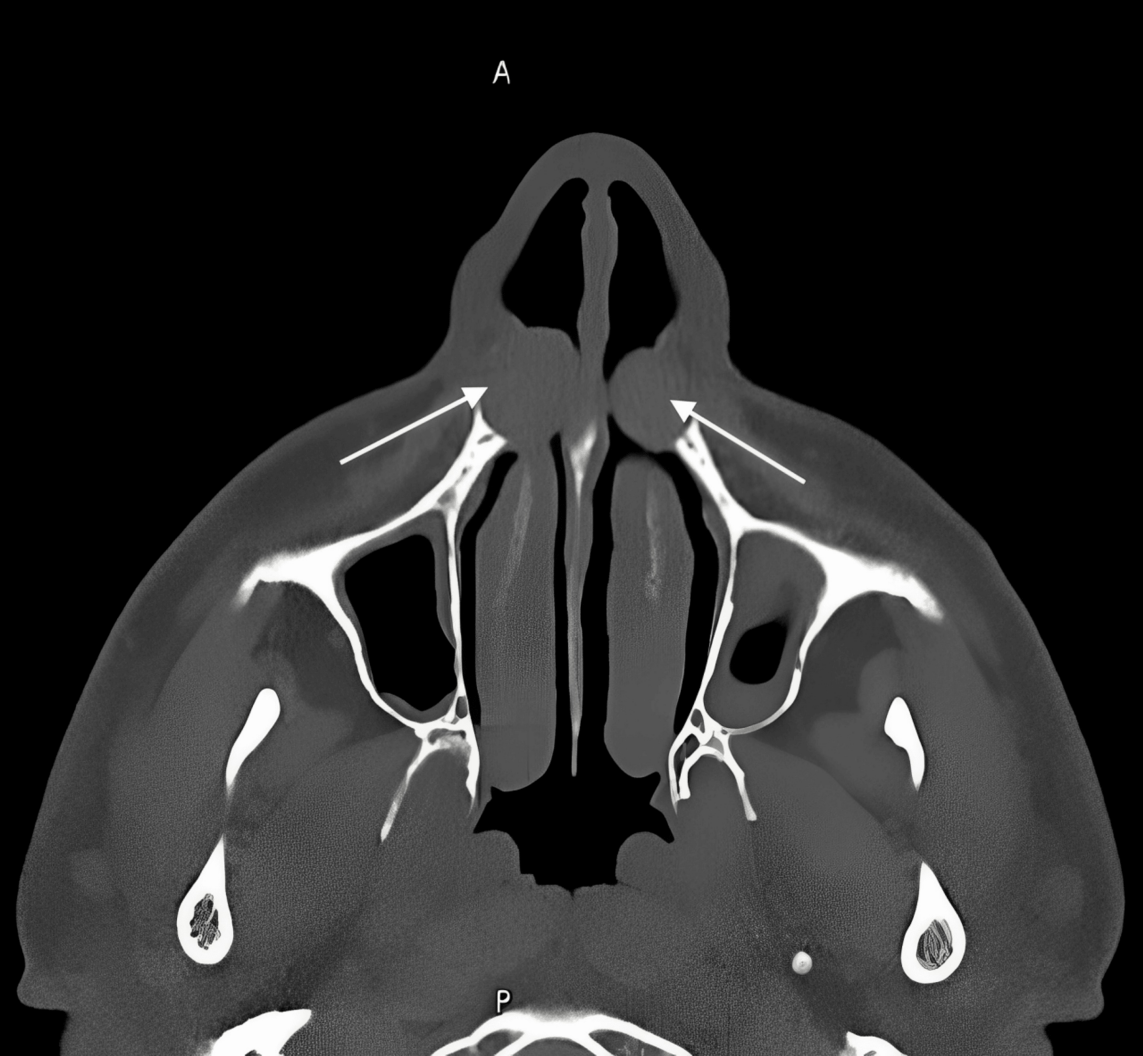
Axial CT of the anterior nasal cavity (performed on June 6, 2024) showing bilateral, well-circumscribed, low-attenuation submucosal lesions along the anterior nasal floor
